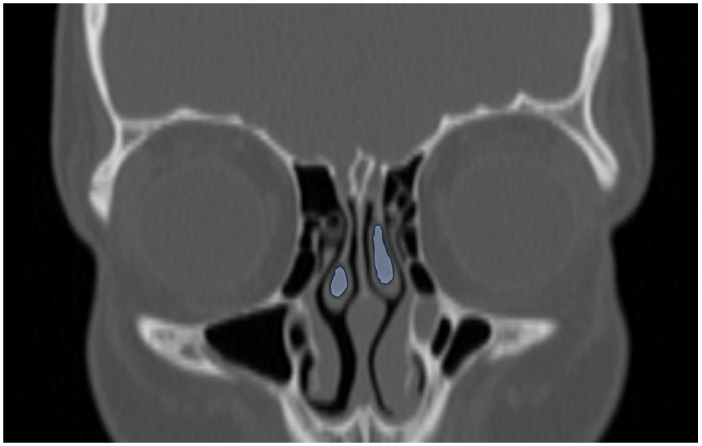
Coronal reformatted CT image of a 12-year-old boy demonstrating bilateral concha bullosa in the middle turbinate (blue areas).
